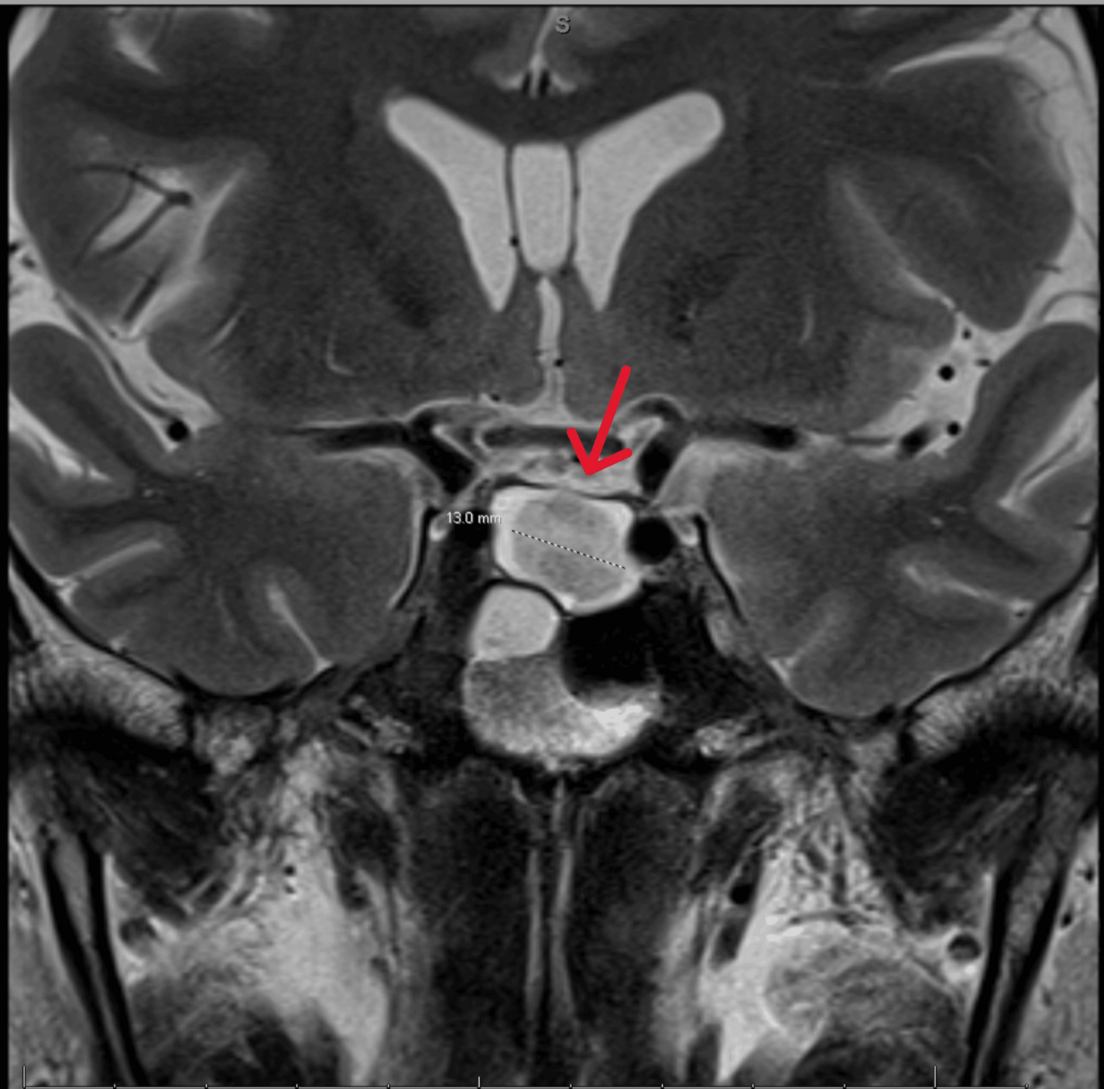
MRI pituitary with and without contrast showing a suprasellar mass in the posterior pituitary Posterior pituitary mass (red arrow) measuring 1.6x1.6x1.7 cm with displacement of the infundibulum and anterior pituitary gland. There is a mass effect on the infundibulum. Differential diagnosis includes craniopharyngioma, neurosarcoidosis versus pituitary apoplexy.
